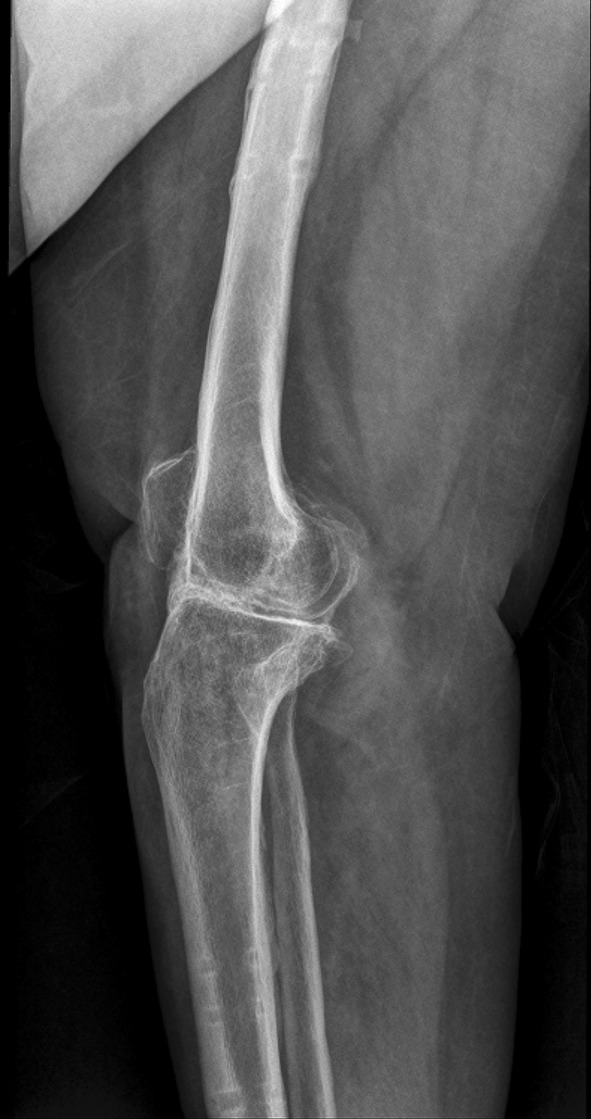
Lateral plain radiograph at seven months showing good consolidation at the arthrodesis site
